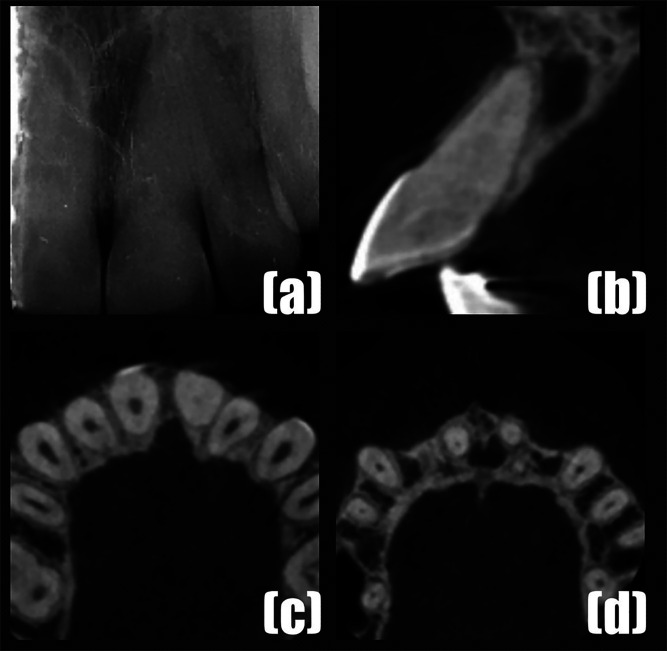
(a) The periapical radiography which revealed PCC and periapical radiolucency in relation to teeth #21. (b-d) CBCT showing PCC of tooth #21.
